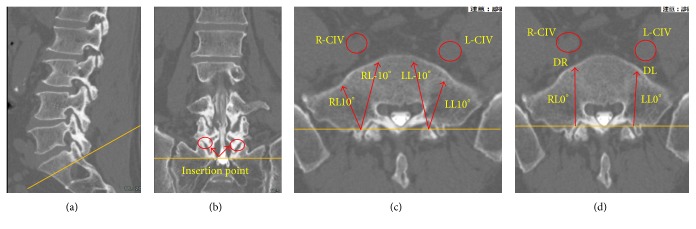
Sagittal coronal and axial computed tomography images from the trajectory parallel to S1 endplate. Abbreviations are as defined in Figure 2.
